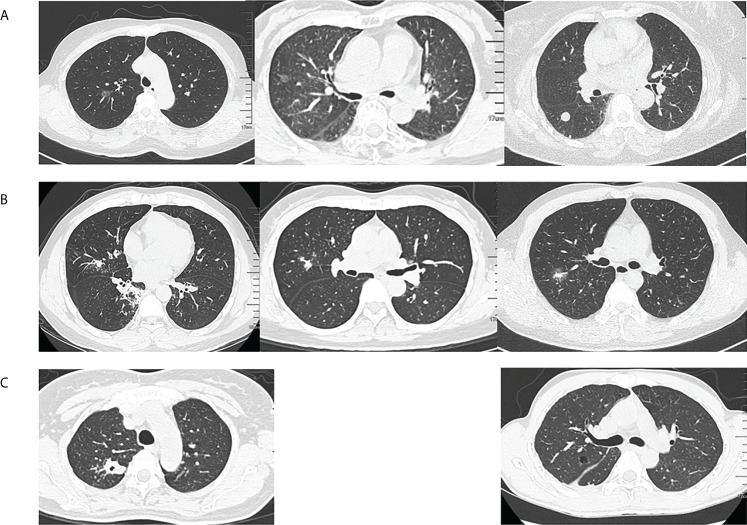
Classification of chest computed tomography (CT) in non-tuberculosis patients. (A) Nodular type: ground glass nodules or solid nodules, (B) Pneumonia type: focal or patchy mixed density shadow, and (C) Mixed type: other manifestations such as cavity, cystic or pleural effusion.
